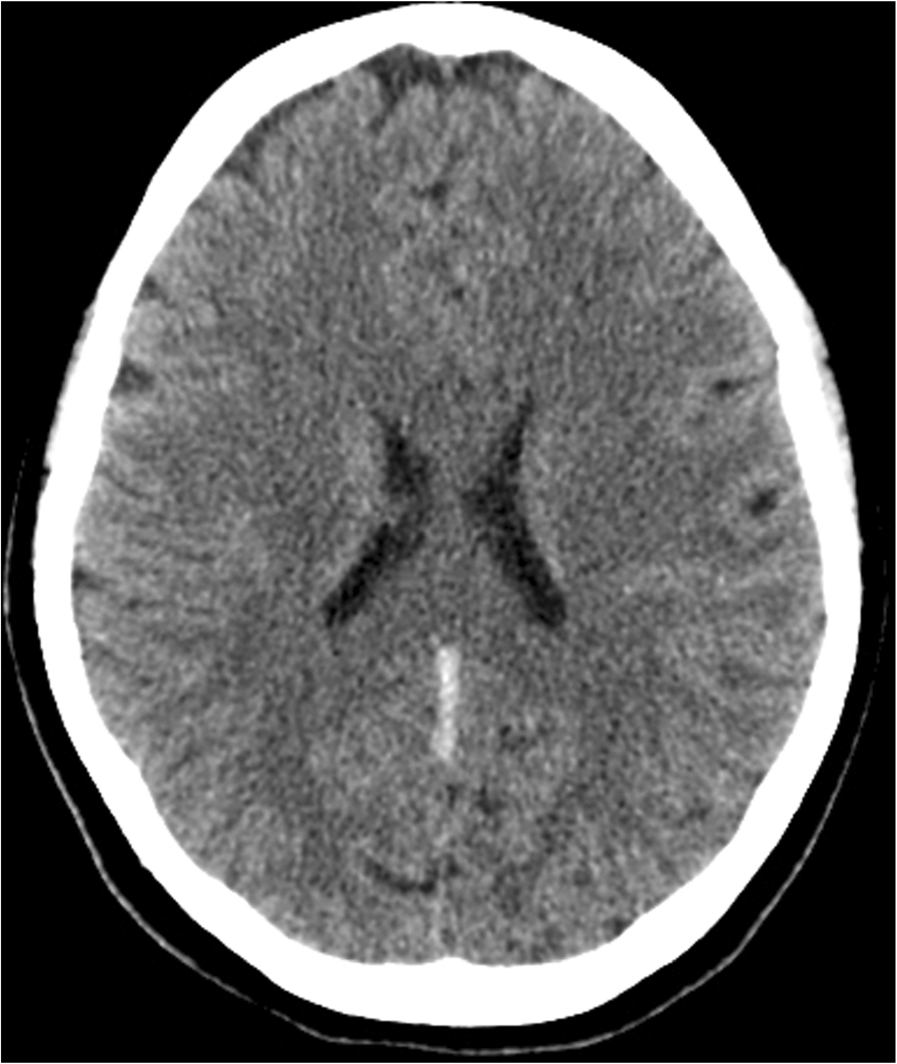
The filling defect in the computed tomography scan with contrast
